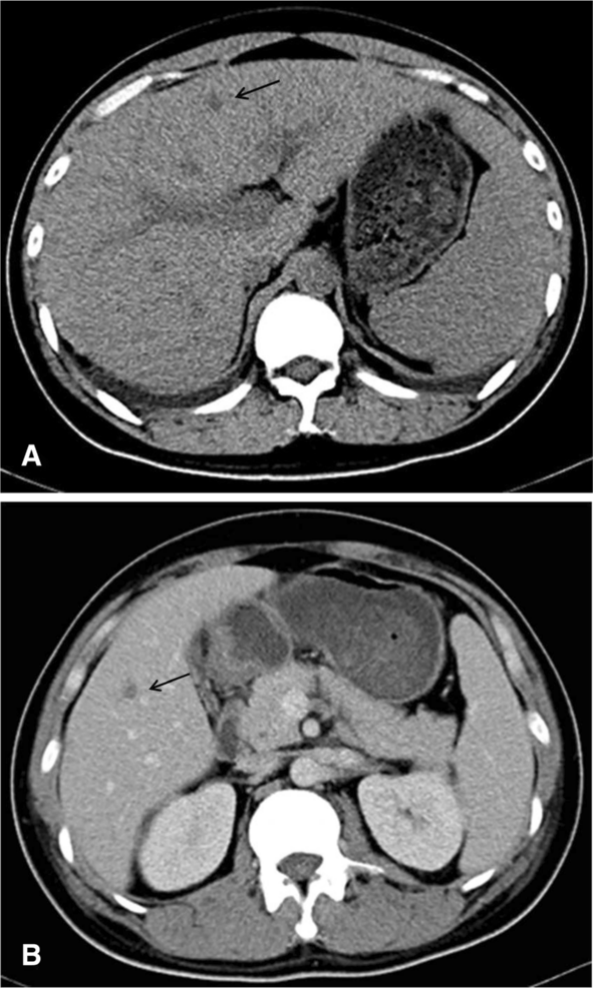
Pre-contrast CT images showing multiple low-density hepatic masses with hepatosplenomegaly and bilateral pleural effusion (a). Post-contrast CT images showing contrast enhancement in the portal venous phase (b)
